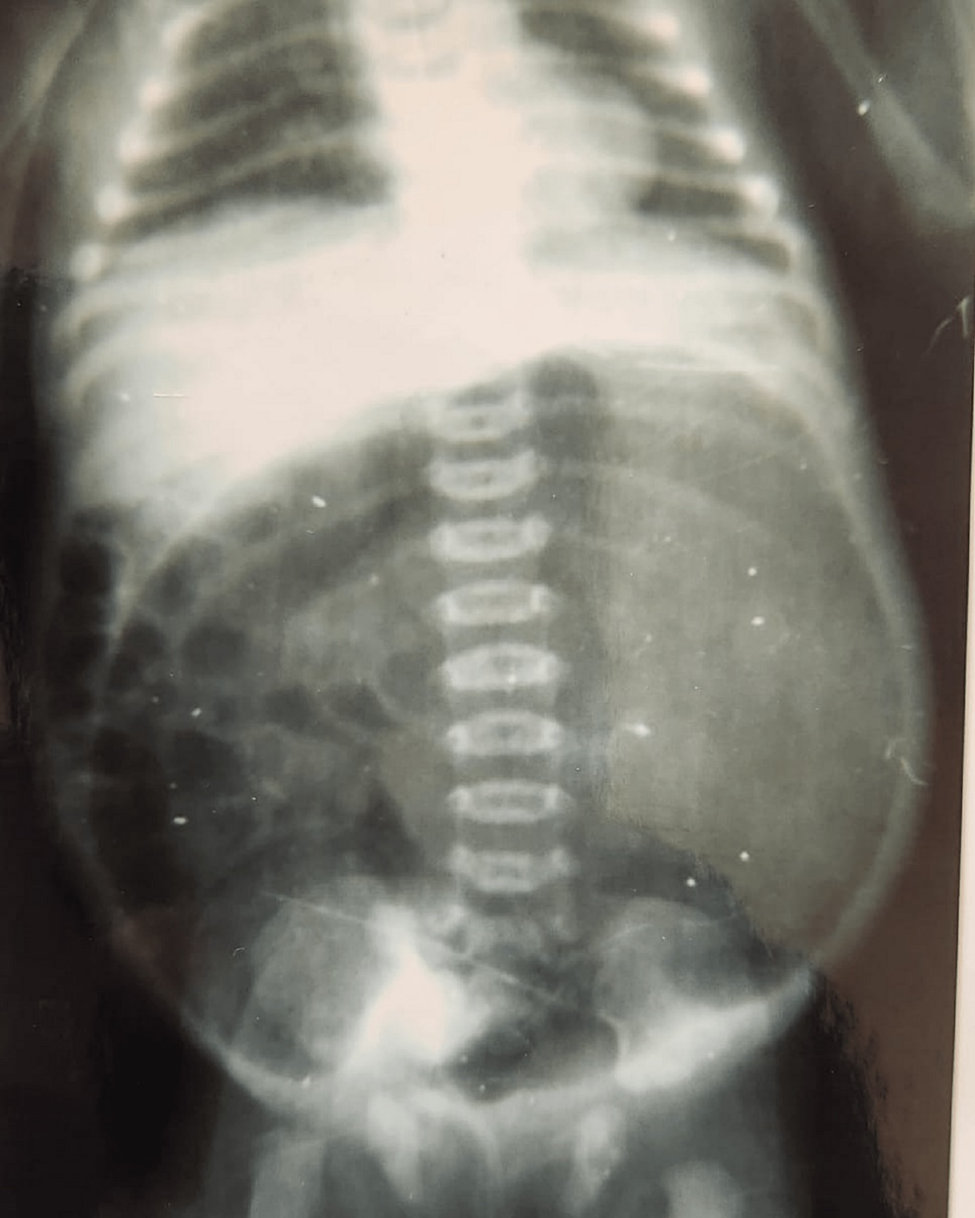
X-ray of the Abdomen With Pouch Colon
